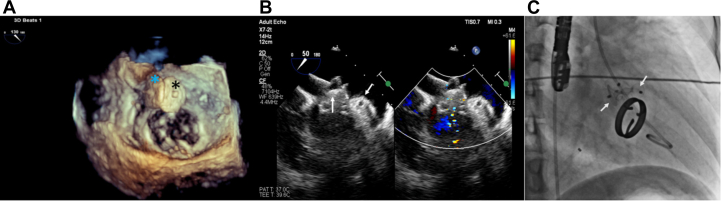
Post-Deployment TEE and Fluoroscopic Images (A to C): Post-deployment transesophageal echocardiography (TEE) and fluoroscopic images. (A) Both the KONAR-MF VSD occluder devices (blue and black asterisks) are shown. (B) TEE midesophageal 50° view with color Doppler, indicating absence of paravalvular leak. Arrows indicate the positions of both paravalvular devices. (C) Fluoroscopic image (RAO 30° view) of both KONAR-MF VSD occluder devices.
